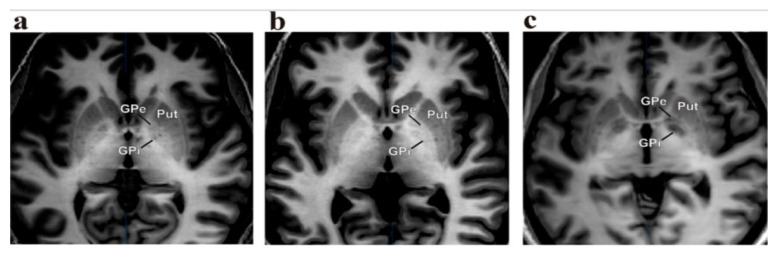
(a–c) A high-resolution T1-weighted, inversion recovery (IR)-weighted contrast-enhanced MRI used to obtain better clarity of the GPi nucleus with GPe and putamen.
